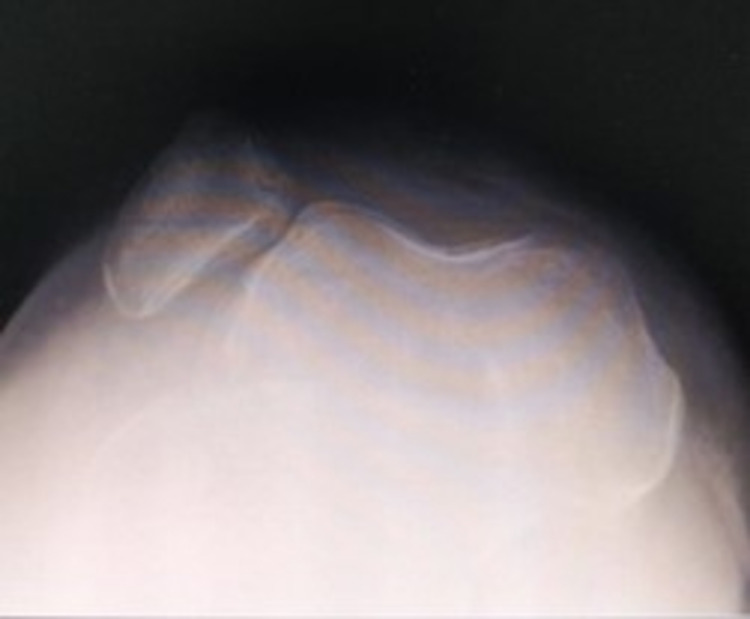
X-ray showing the axial view of the patella.
